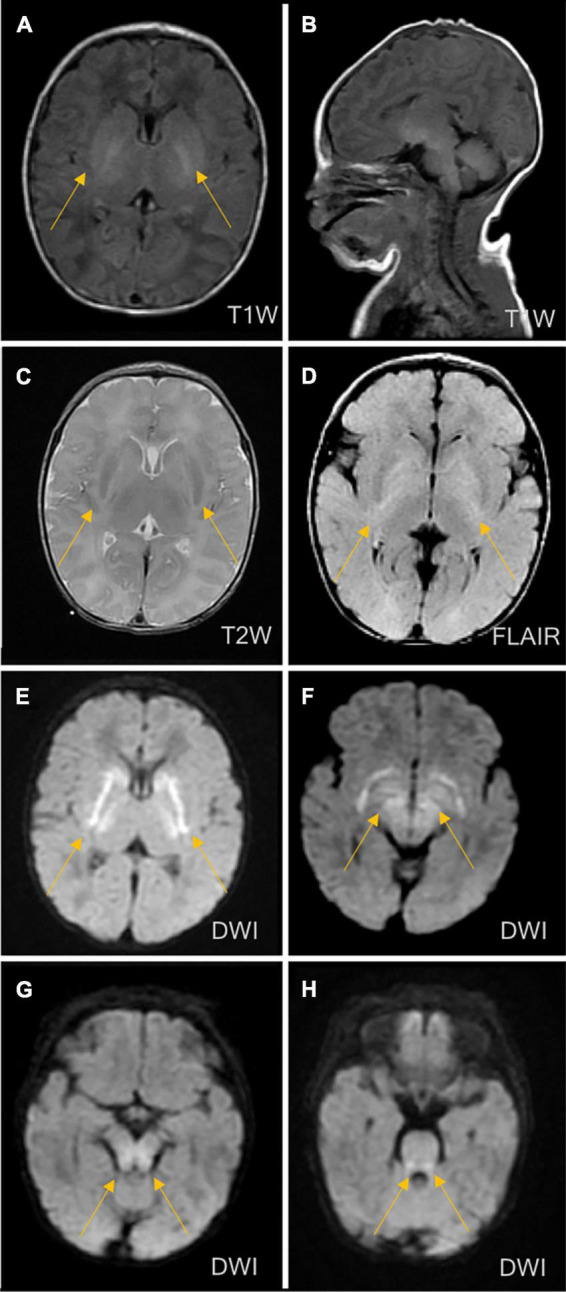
Brain magnetic resonance imaging (MRI) on the third day of life. MRI showed symmetrical diffuse high-signal shadows in the bilateral basal ganglia, cerebellum, pontine, tegmental and bilateral frontal and parietal cortices, suggesting hereditary metabolic leukoencephalopathy. (A) No abnormal signal intensity in the bilateral basal ganglia on the T1-weighted image (T1WI). (B) Low signal intensity in the bilateral basal ganglia on the T2-weighted image (T2WI). (C) High signal intensity in the bilateral basal ganglia on the diffusion-weighted image (DWI). (D) High signal intensity in the bilateral basal ganglia on fluid-attenuated inversion recovery (FLAIR). (E) High signal intensity in the cerebellum on the DWI. (F) High signal intensity in the pontine on the DWI. (G) High signal intensity in midbrain on DWI. (H) High signal intensity in medulla on DWI.
